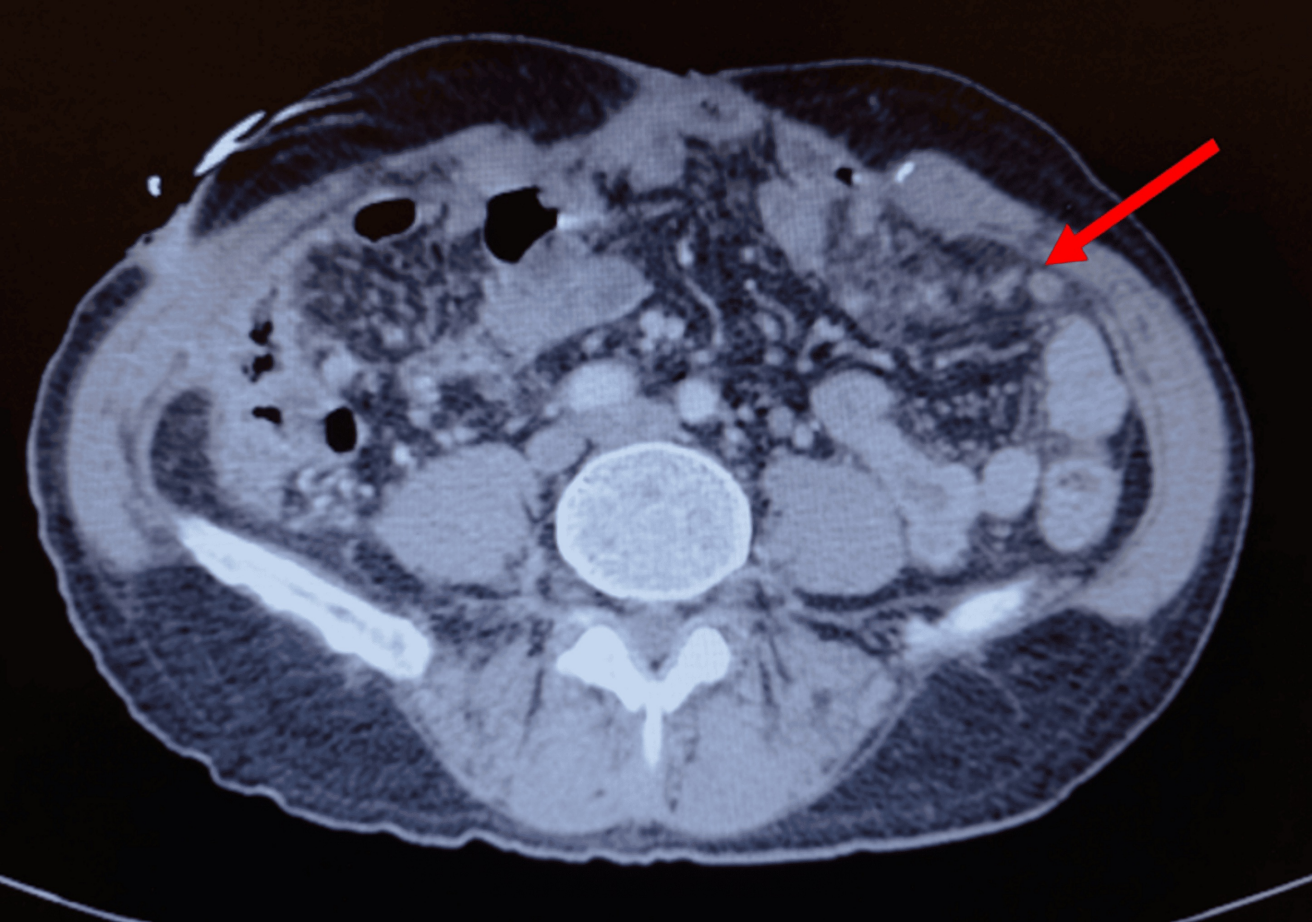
Contrast-enhanced CT scan (axial view) of the abdomen six weeks after surgery showing diffuse peritoneal carcinomatosis (red arrow)
